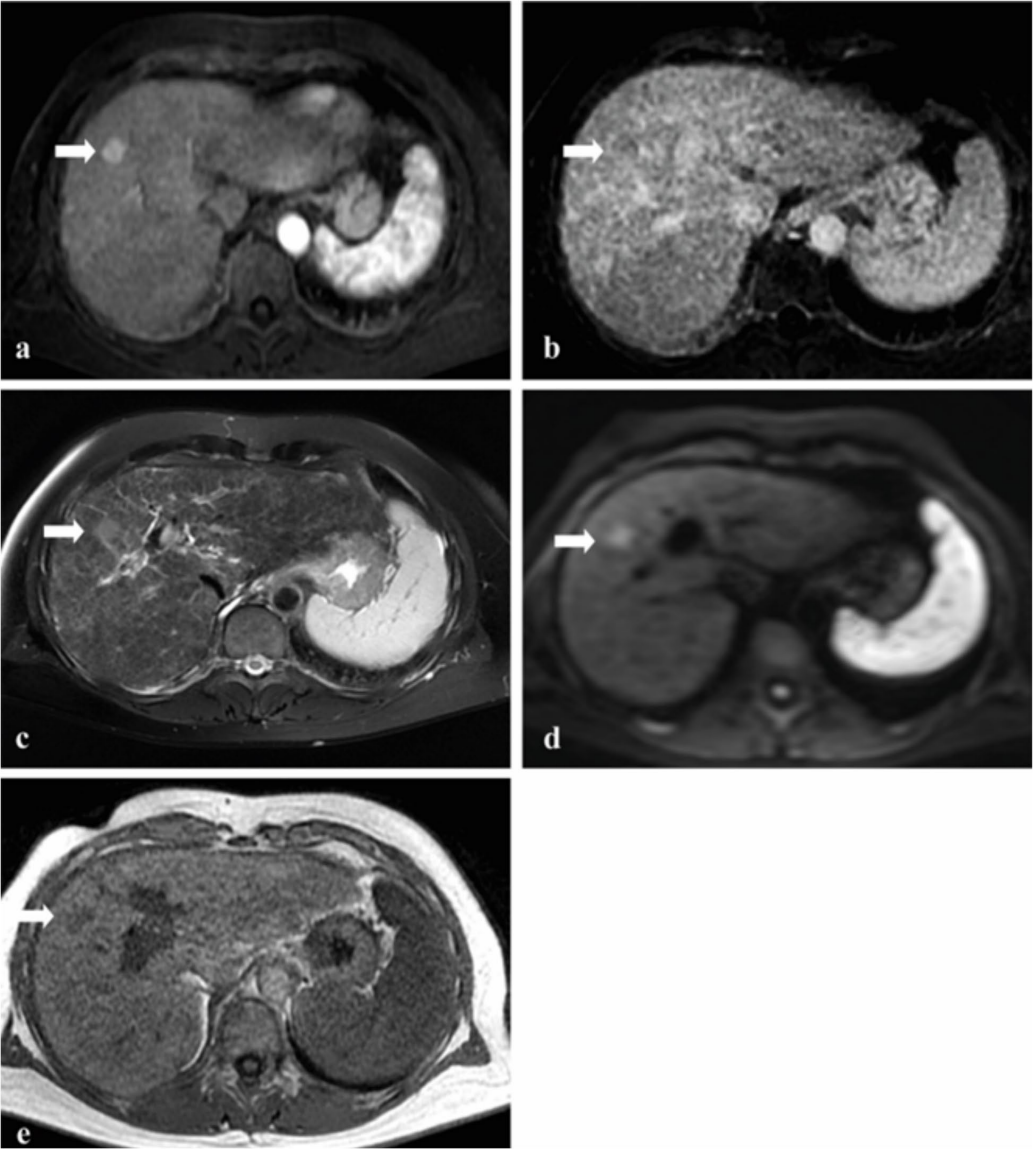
Images of a patient with chronic hepatitis C cirrhosis using the full MRI liver protocol. Newly found hypervascular lesion (arrows) measuring 13 mm in diameter can be seen (a), showing wash-out (b) and hyperintensity on T2-weighted image (c), as well as hyperintensity on diffusion-weighted image (d) and hypointensity on T1-weighted image (e). The lesion was classified as LI-RADS 5
